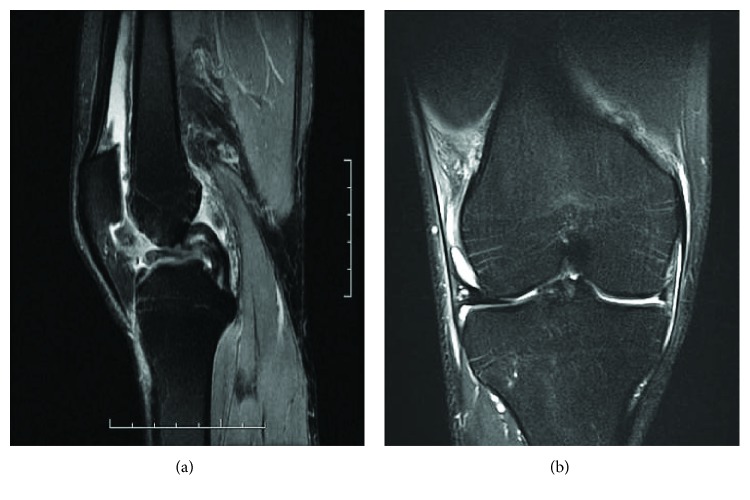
Magnetic resonance imaging in T2 of the knee with sagittal and coronal sections demonstrating the bucket handle tear of the medial meniscus.
